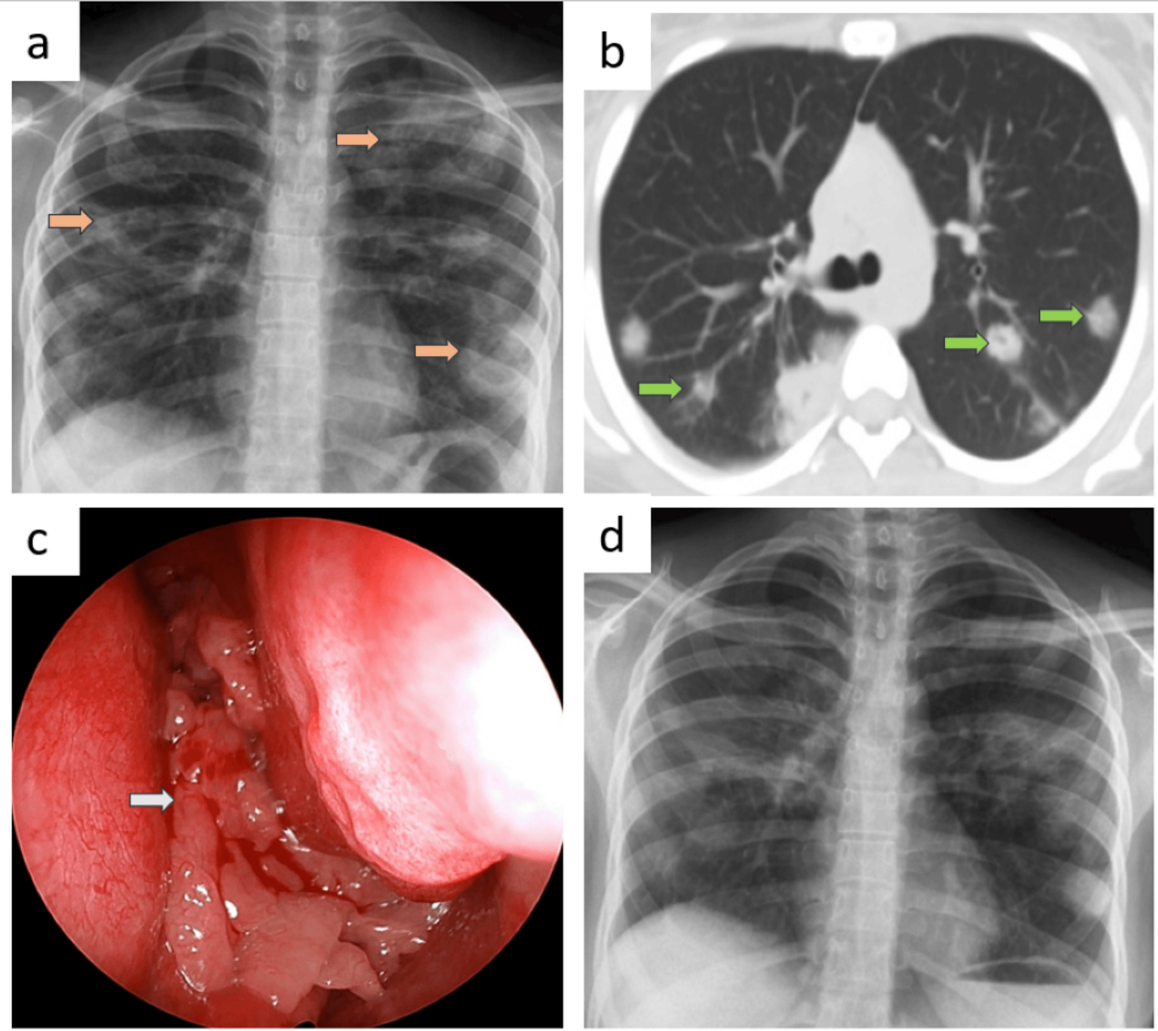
Radiology and anterior rhinoscopy images of patient A at diagnosis and during follow-up. (a) Chest X-ray imaging indicated bilateral rounded non-homogenous opacities across all lung zones marked by brown arrows. (b) Computed tomography (CT) revealed bilateral multilobar lung consolidation and cavitating nodules marked by green arrows. (c) Anterior rhinoscopy examination showed multiple glistening cluster of grapes-like lesions suggestive of left nasal polyposis marked by a white arrow. (d) Follow-up chest radiography after four months of treatment showed resolution of lesions.
